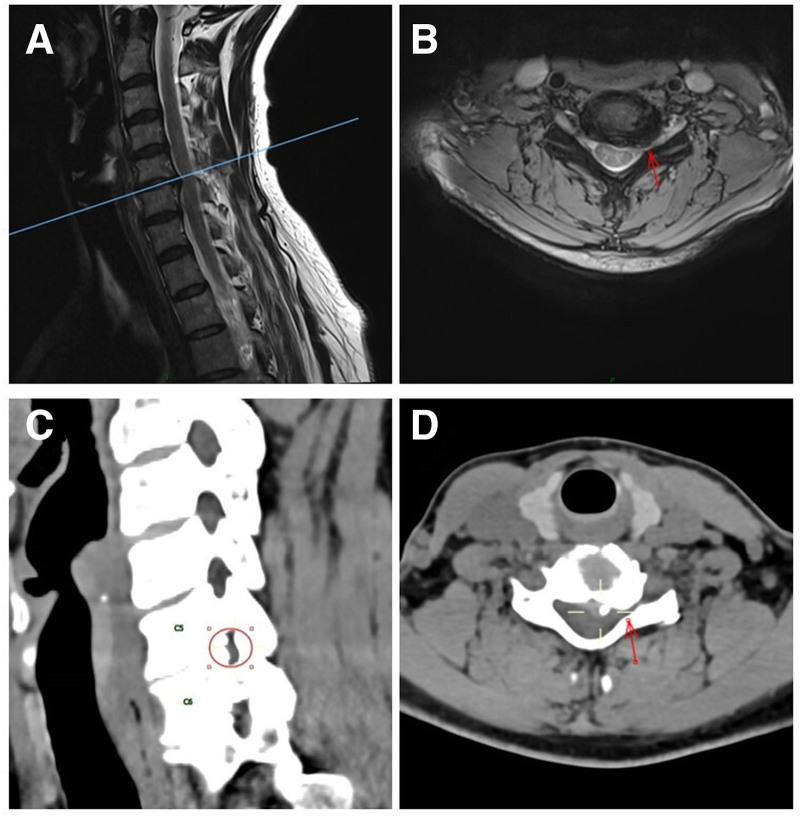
Preoperative T2W1 MRI imaging of the patient’s sagittal (A) and axial (B) reveals left-sided foraminal stenosis and the C5–C6 intervertebral disc herniation to the left rear. The preoperative CT examination (C, D) indicated stenosis of the 5 to 6 cervical foramen with uncovertebral joint hyperplasia. CT = computerized tomography, MRI = magnetic resonance imaging.
